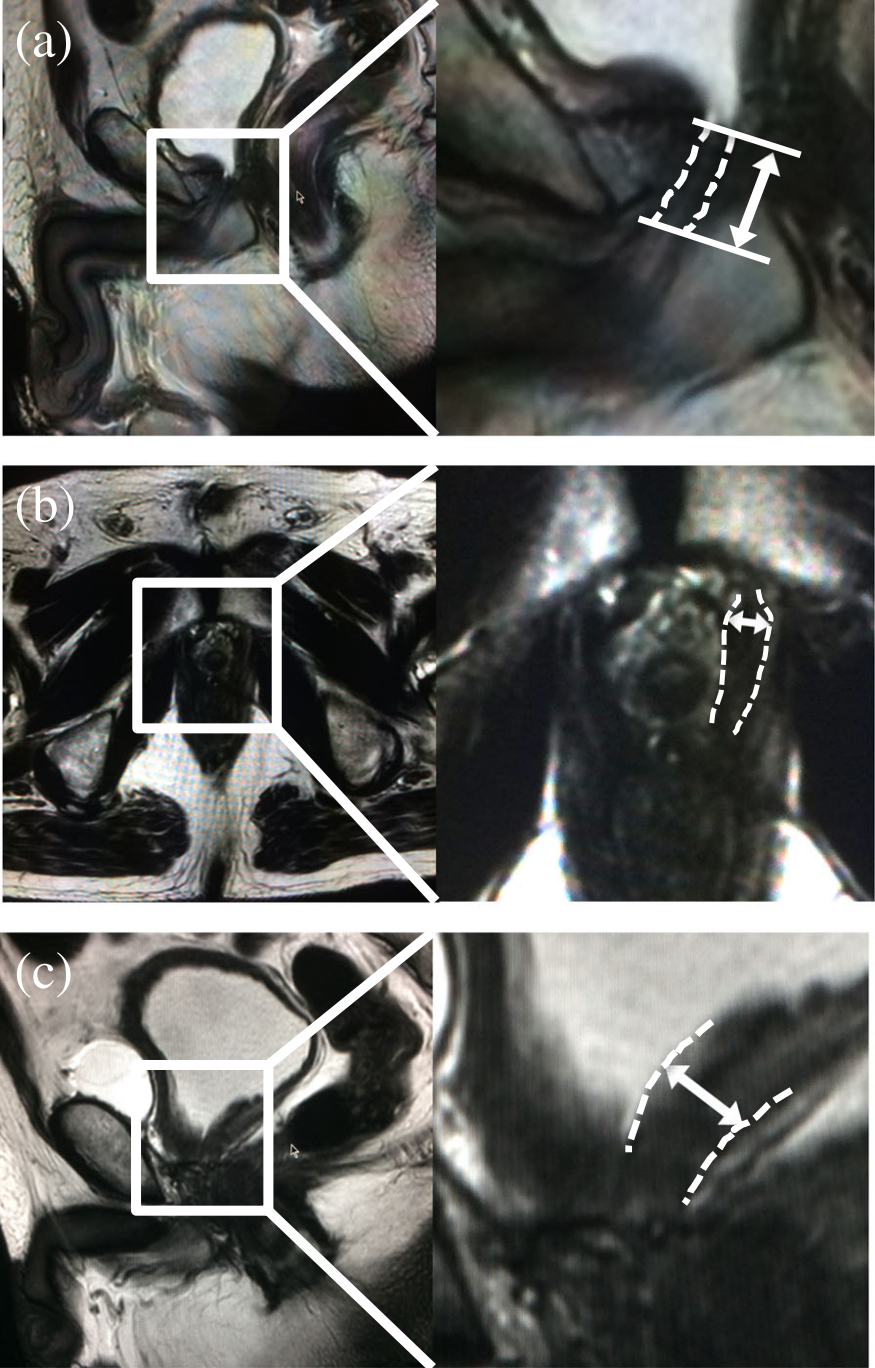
Postoperative pelvic anatomic features on T2-weighted magnetic resonance imaging. a Membranous urethral length measured in the sagittal plane. The dashed lines show the membranous urethra. b Levator muscle width measured in the axial plane. The dashed lines show the levator muscle. c Bladder neck width on the trigone side measured in the sagittal plane. The dashed lines show the posterior bladder neck wall
